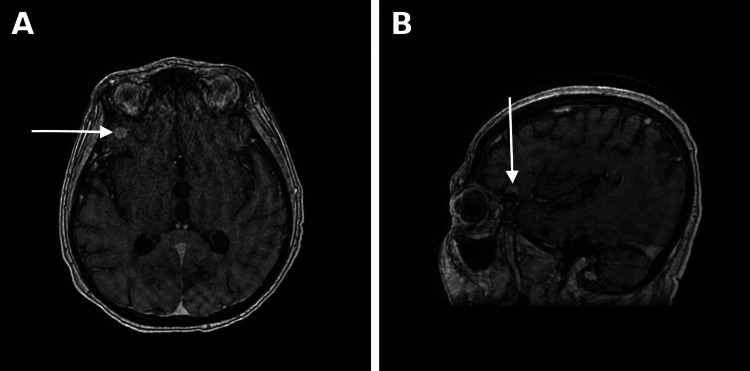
MRI of the brain with and without IV contrast demonstrating a 7 mm extra-axial enhancing mass with a broad dural base along the right greater wing of the sphenoid (arrows), with imaging features suggestive of meningioma versus dural metastasis. (A) Axial view. (B) Sagittal view.
